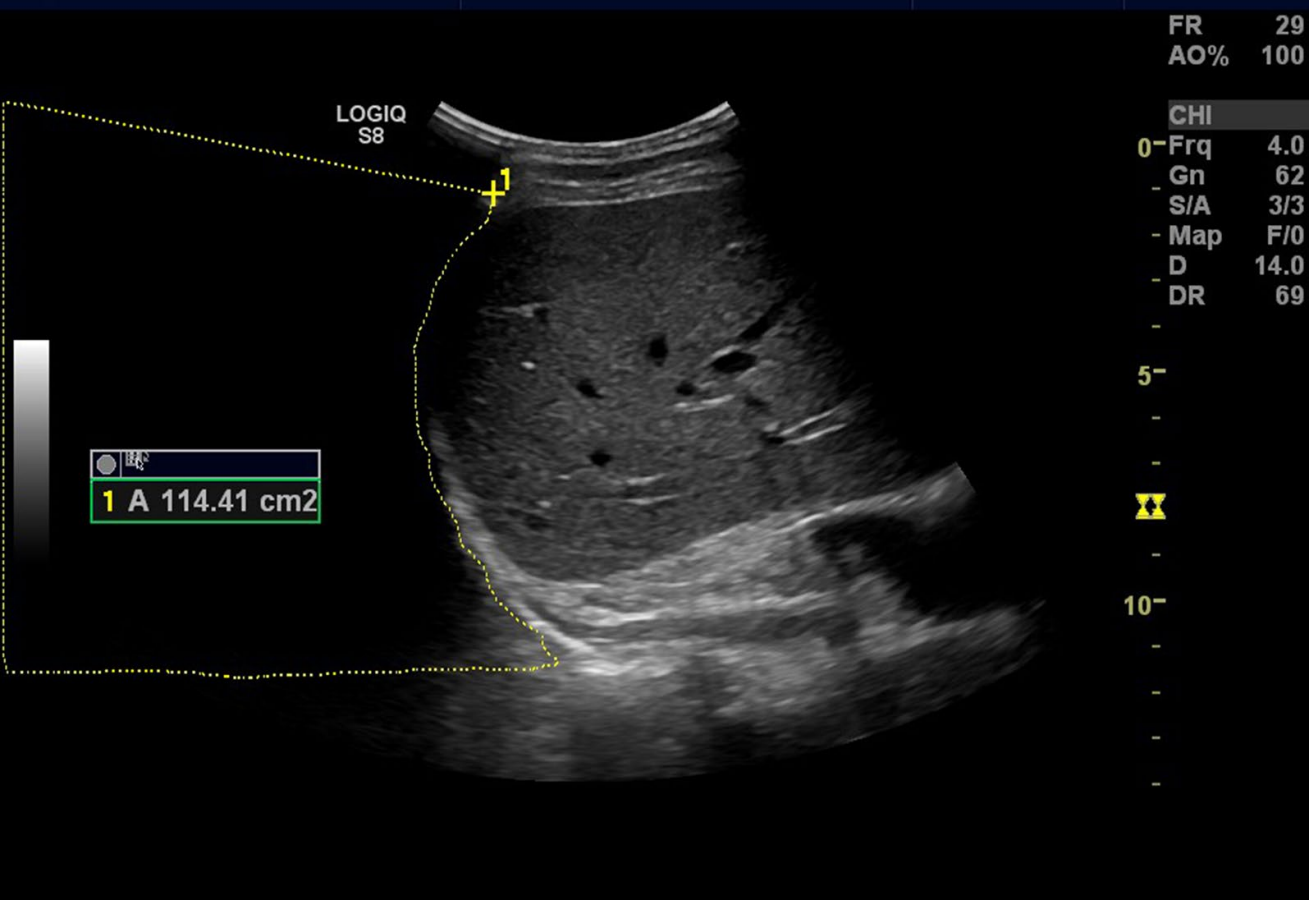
Lateral ultrasound view in the mid-axillary line. Diaphragm is identified behind the liver. With the ultrasound machine’s inbuilt area function the area over the diaphragm is measured in maximal inspiration
